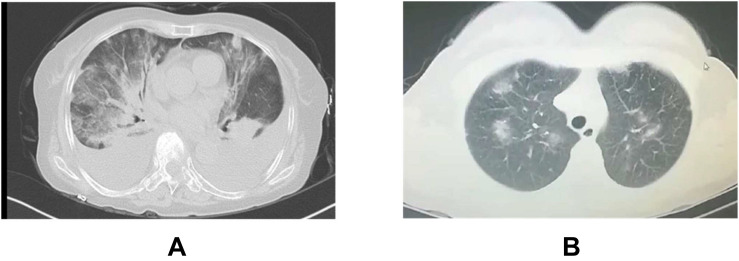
Typical CT of TKIs-pneumonitis (A) and viral pneumonitis (B) A: Female, 65 years old, advanced lung adenocarcinoma, TKI after 3 months in our cancer hospital B: Female, 42 years old, outpatient CT scanning for cough in our cancer hospital.
